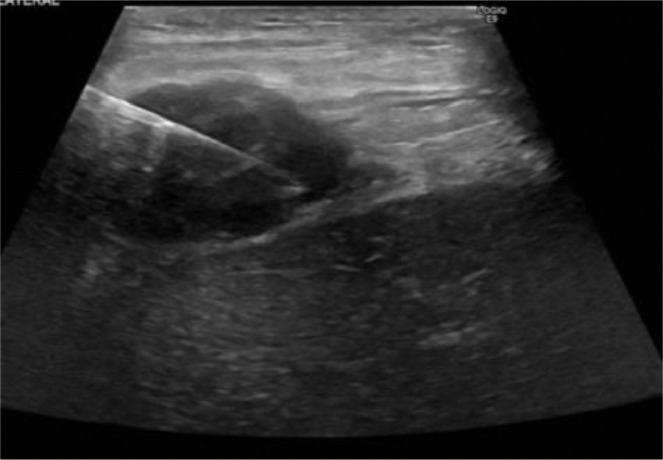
Ultrasound guided biopsy of right sided pleural thickening with core biopsy needle.
